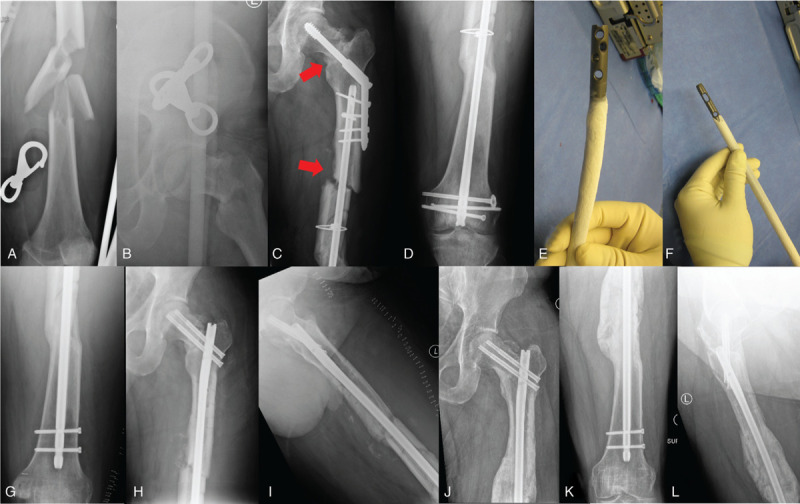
Femoral neck-shaft combined fracture nonunion. Radiographs of a 42-year-old male who presented with an open left femoral shaft fracture and closed femoral neck fracture after a motorcycle collision (A and B). He was initially treated with a retrograde intramedullary nail and dynamic hip screw (DHS) construct, which developed a nonunion (C and D). His radiographs demonstrate nonunion of his femoral shaft as well as his femoral neck with varus malalignment of both fractures (red arrows). His preoperative workup revealed normal ESR and CRP and a low vitamin D level, which was corrected prior to surgery. He was taken to the operating room for complete hardware removal, irrigation and debridement, revision fixation with an antibiotic coated cephalomedullary nail (with correction of varus alignment), and placement of platelet-derived growth factor (PDGF, Augment, Wright Medical) at the nonunion site (E–I). Three of 5 deep cultures were “surprise positive” (2/5 for *staphylococcus epidermidis* and 1/5 for *propionibacterium acnes*). He was treated with 6 weeks of postoperative IV antibiotics and 6 weeks of oral antibiotics. Nine-month postoperative radiographs show solid union and the patient was clinically free of infection (J–L).
